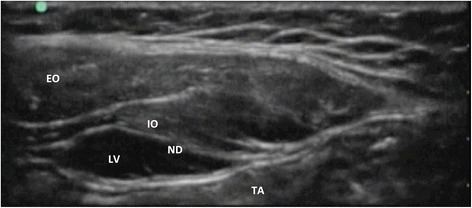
TAP block was performed bilaterally with ultrasound echo. Three layers of muscles forming the anterior abdominal wall, from superficial to deep: external oblique (EO), internal oblique (IO), and transversus abdominus (TA). A 22-G Tuohy block needle (ND) was advanced directly towards the TA. Levobupivacaine (LV) (0.25 %) was administered at a total of 10 ml on each side of TA
